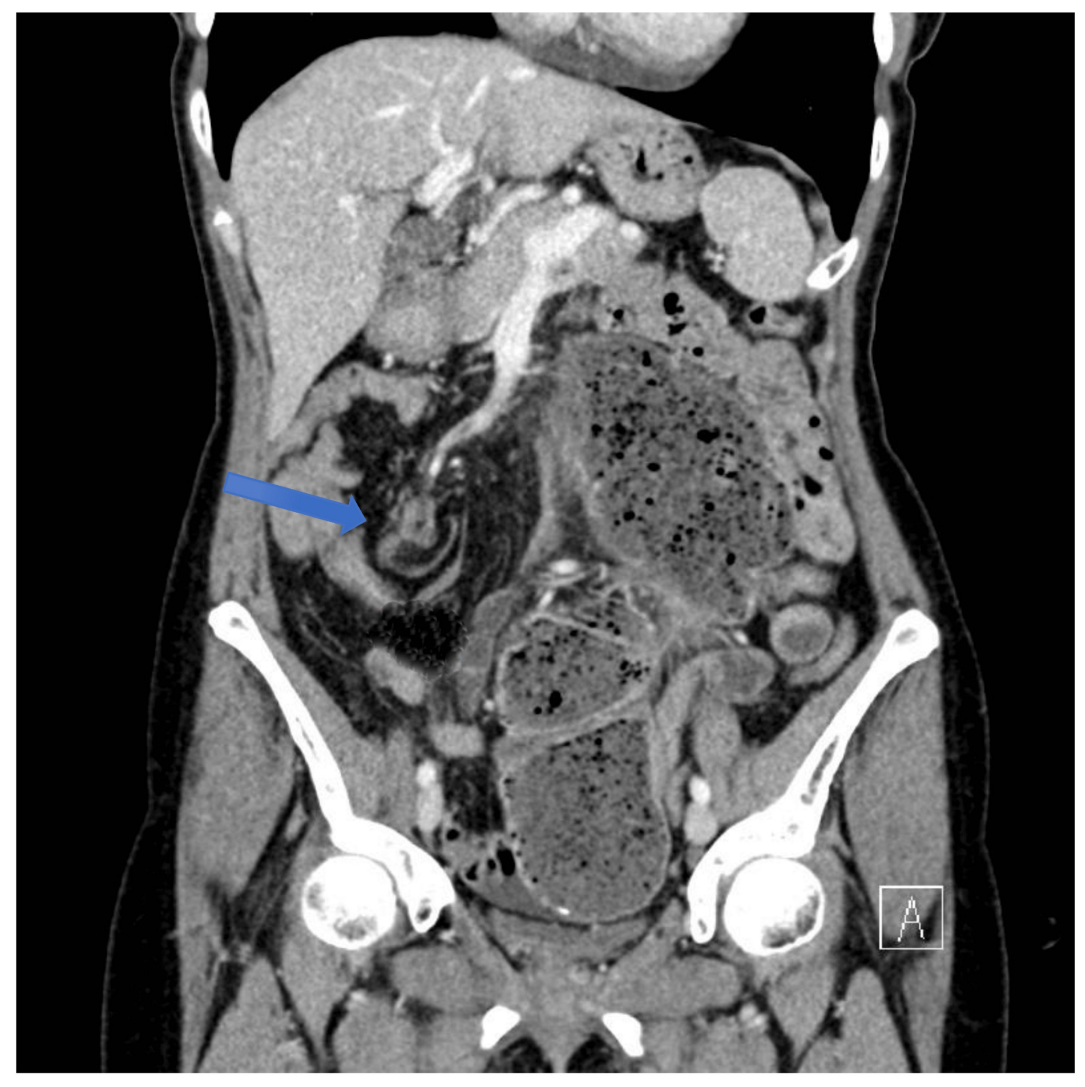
CT scan of abdomen and pelvis, showing cecal volvulus The blue arrow points to whirls, indicating twisting of cecal mesentery.
